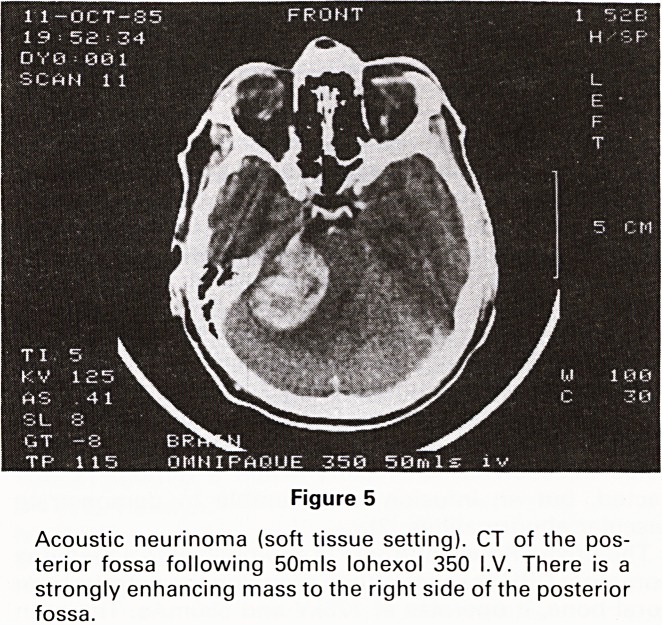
# Computed Tomography of the Internal Auditory Canals
